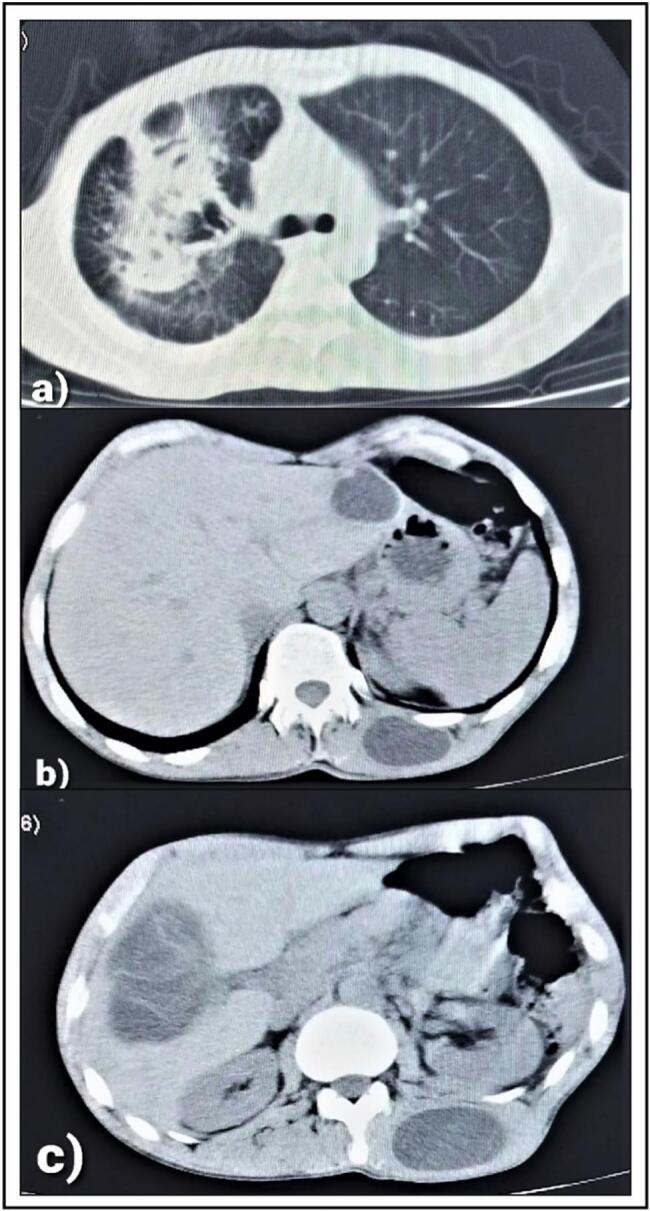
CT scan reveals: (A) two open cysts in the right lung. (B) 4 cm cyst in the left hepatic lobe and the cyst in paraspinal muscles on the left side of the patient. (C) 8 cm cyst in the right hepatic lobe and the cyst in paraspinal muscles on the left side of the patient.
